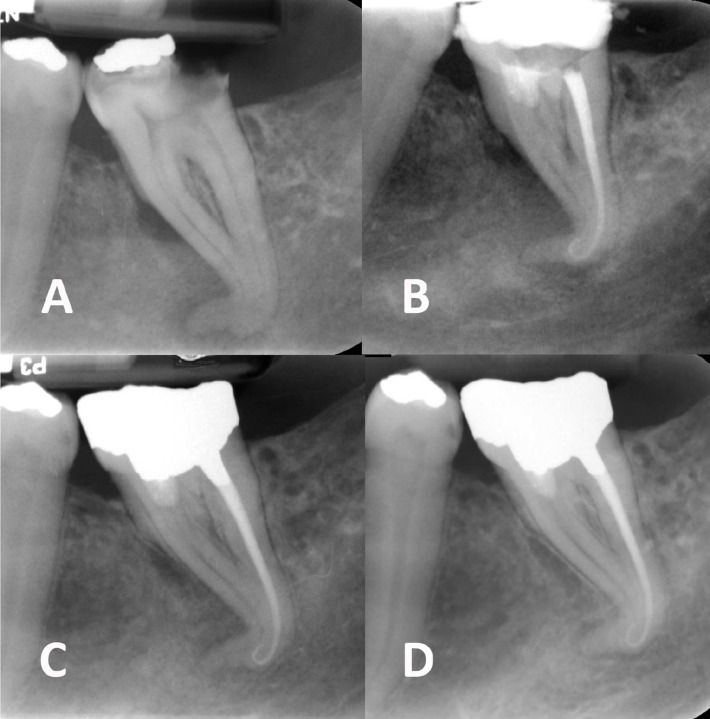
A) *Pre-operative periapical radiography;* B) *Post-operative image; *C-D) *Short- and long-term (12-month) follow-up images
